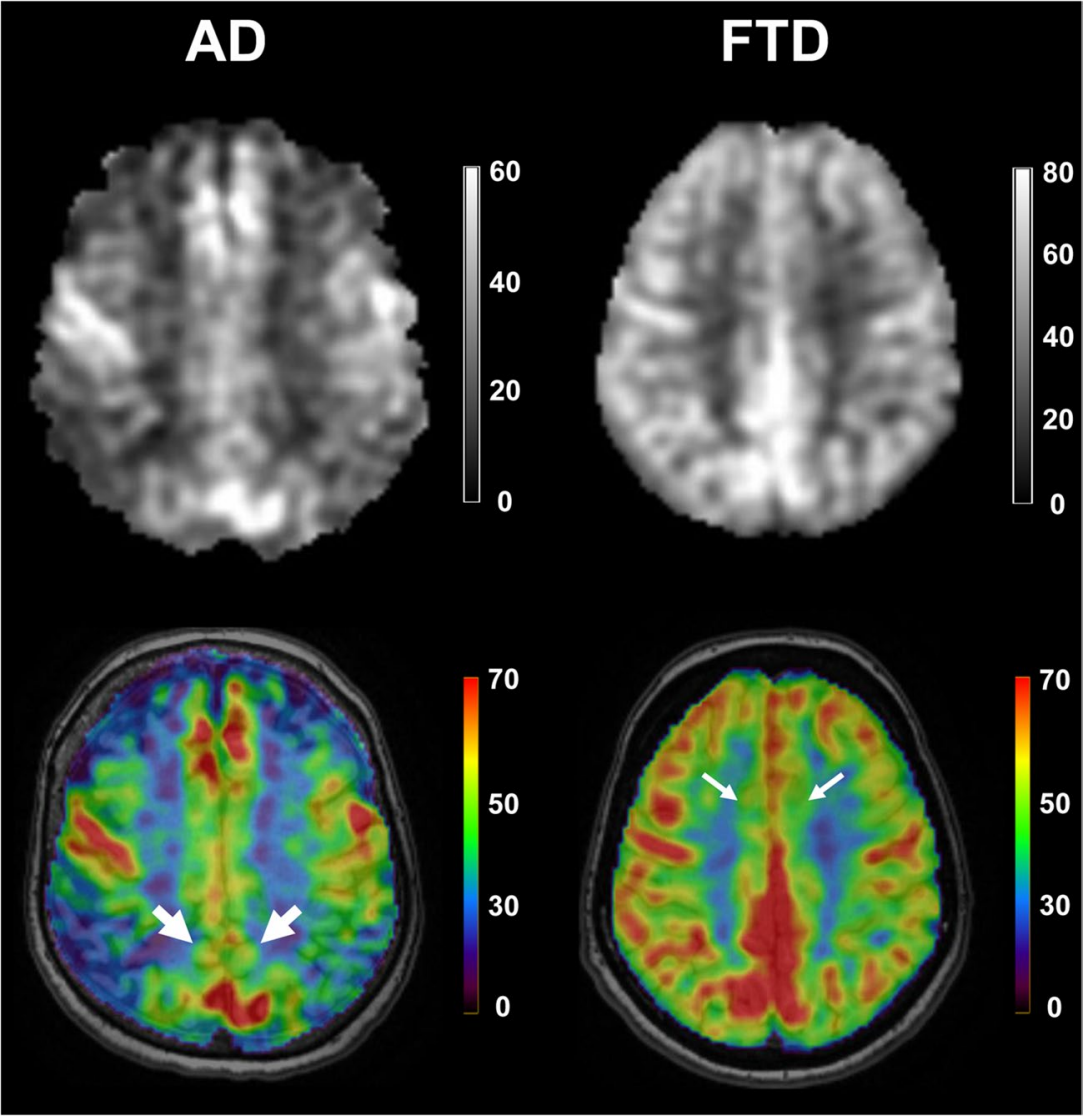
Cerebral blood flow maps of one patient with Alzheimer’s disease (AD, left column) and one patient with frontotemporal dementia (FTD, right column). The bottom row shows colour-coded cerebral blood flow maps coregistered onto high-resolution T1-weighted magnetic resonance images. Please note the occurrence of prominent hypoperfusion in the precunei and adjacent posterior cingulate cortices (thick arrows) of the patient with AD, as well as focal hypoperfusion in the anterior cingulate cortices of the patient with FTD (thin arrows). Please also note that the grey scale, not the colour scale, is different between patients to optimise the display of relative hypoperfusion. Reproduced under the Creative Commons Attribution 4.0 International License with permission of the authors: Steketee RME, Bron EE, Meijboom R, Houston GC, Klein S, Mutsaerts HJMM, Mendez Orellana CP, de Jong FJ, van Swieten JC, van der Lugt A, Smits M (2016) Early-stage differentiation between presenile Alzheimer's disease and frontotemporal dementia using arterial spin labeling MRI. Eur Radiol 26(1):244–253 [30]
